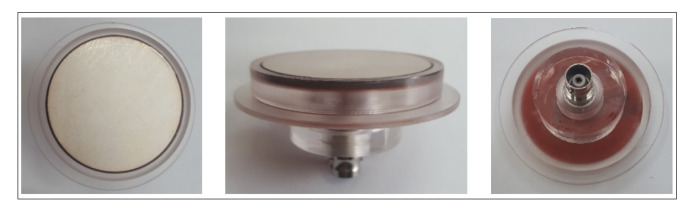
Ultrasonic emitter used.
